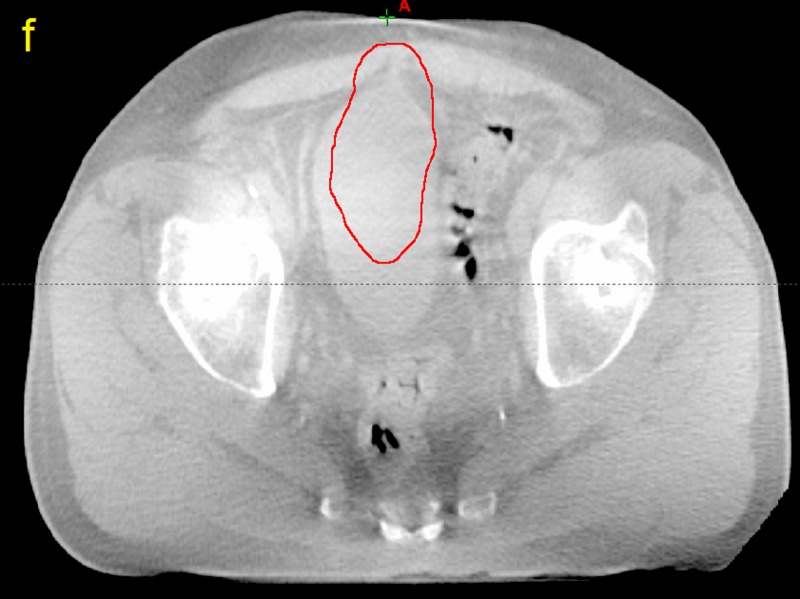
Computed tomographic (CT) image taken from patient P12 f) The planning target volume superimposed on the cone beam computed tomography image
